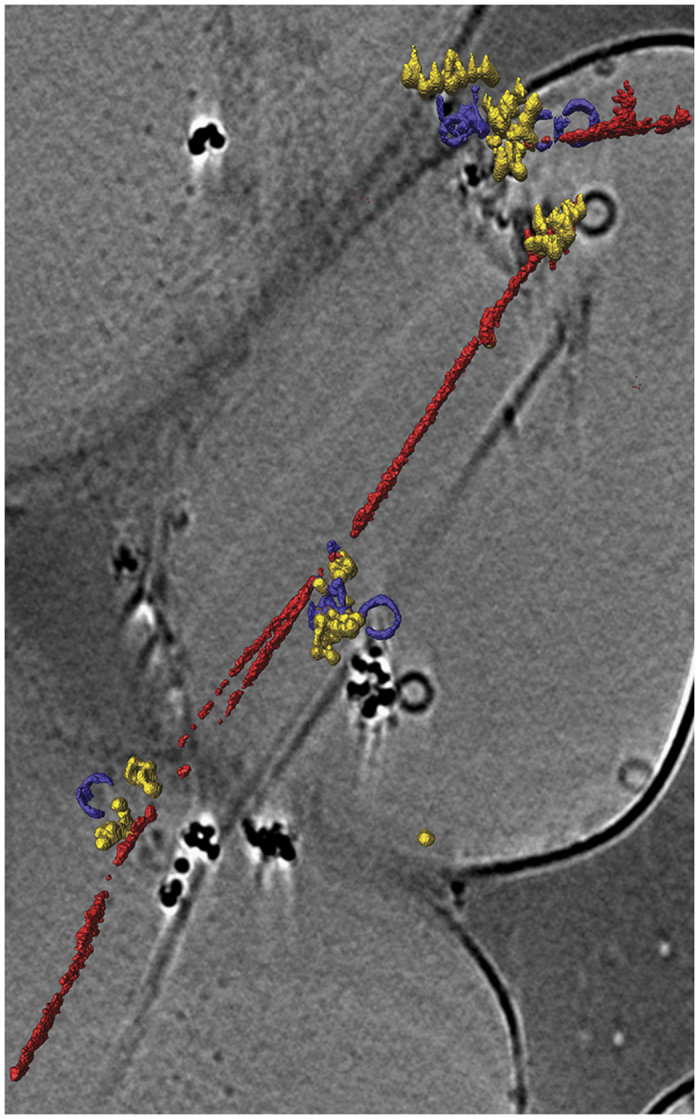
Cryo-EM image and 3D representation of the ternary complex between Btub fibers (red), SUVs (purple) and Bklc + Ni-NTA-Gold (yellow). The 3D iso-surfaces are overlaid on a 2D image corresponding to one z-plane from the tomogram.
